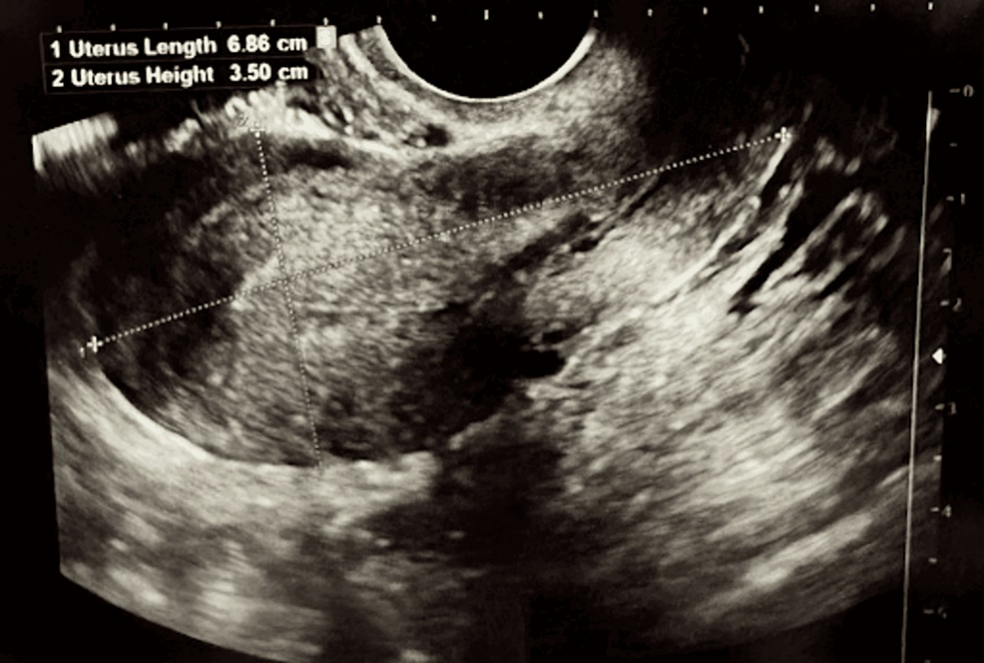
Ultrasound of the patient’s uterus measuring 6.86 x 4.19 x 3.50 cm.
